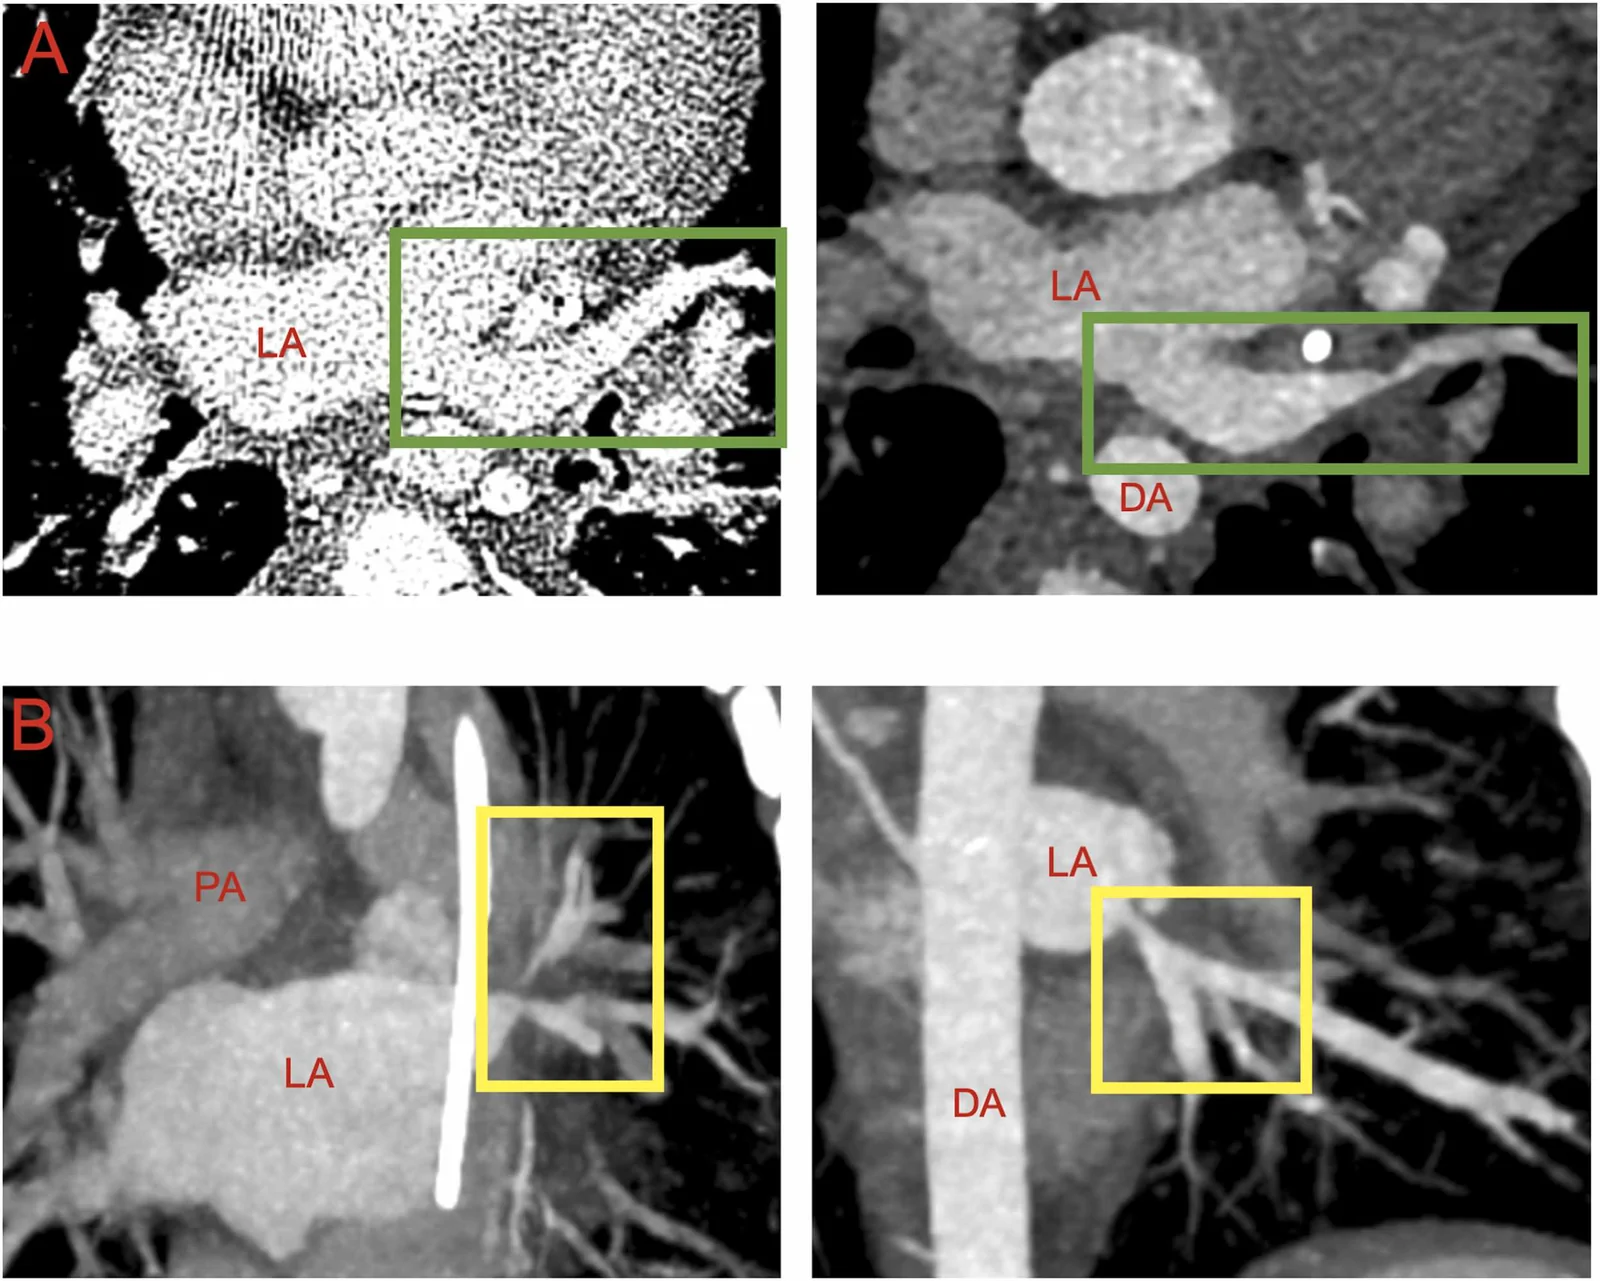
Chest CT findings of recurrent PVS post lung transplantation. (A) Axial views of the Chest CT obtained 11 months after transplant (left) and cardiac CT 34 months after transplantation (right). Note the distal narrowing of the left pulmonary vein highlighted in the green box. (B) Coronal views of the cardiac CT obtained 34 months after transplant, demonstrating stenosis (yellow boxes) of the left upper vein (left) and atresia/stenosis of the left lower vein (right). *DA: descending aorta, LA: left atrium, PA: pulmonary artery.*
